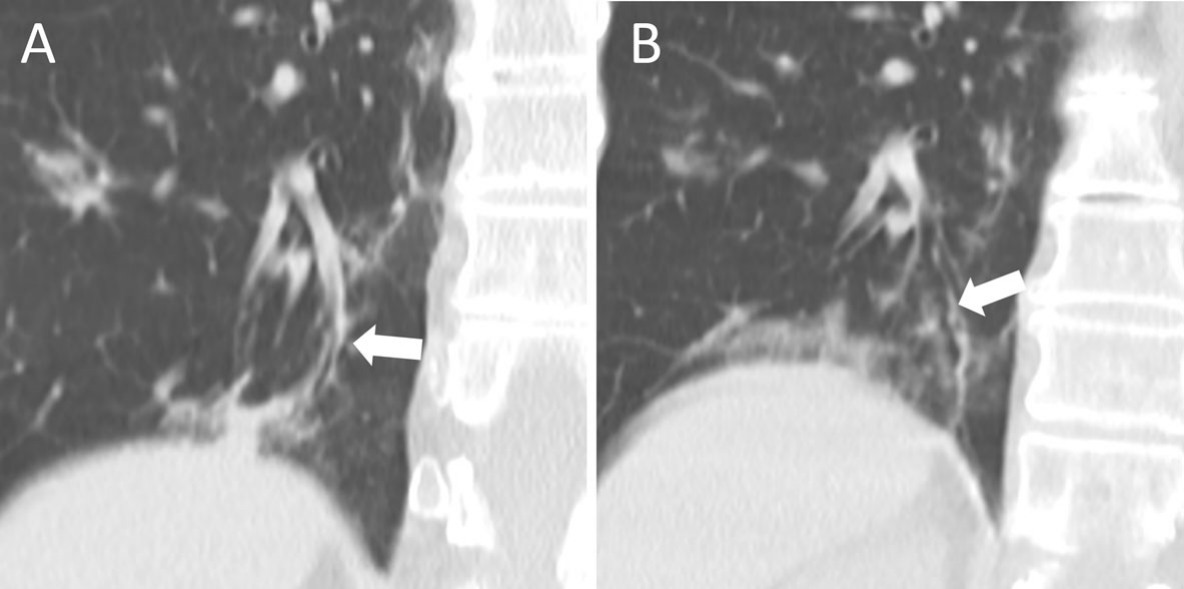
Coronal chest CT images of bronchovascular bundle of COVID-19 pneumonia. Note: (a). Bronchovascular bundle distortion; (b). Bronchovascular bundle distortion reversed. All images have the same window level of − 600 and window width of 1600
